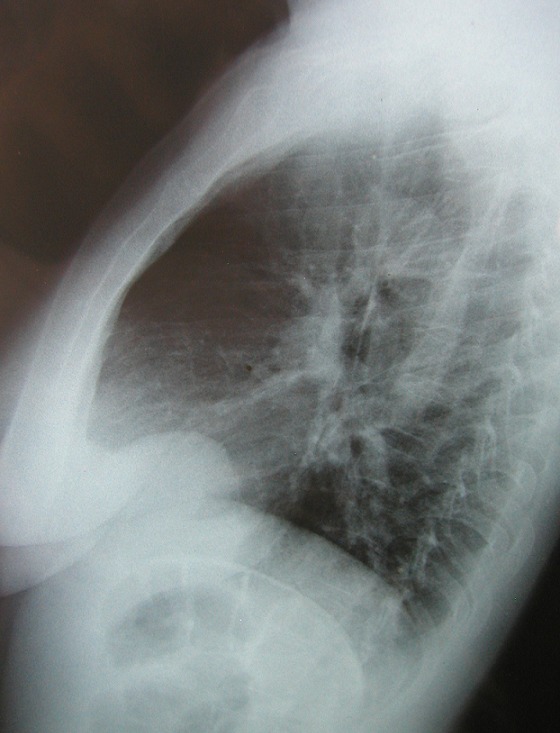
Radiographie thoracique de profile montrant l'aspect d'une surélévation localisée en avant de la coupole diaphragmatique
